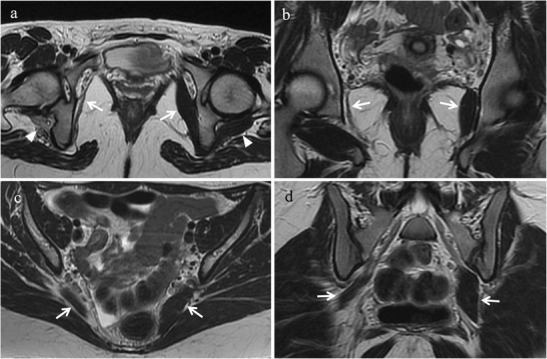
Indirect MR findings of sciatic nerve endometriosis. The same patient as in Fig. 23. (a) Axial and (b) coronal T2-weighted images show atrophy of right obturator internus (white arrows) and gemellus superior muscle (white arrowhead in a) compared with the contralateral ones (white arrows and white arrowhead in a). (c) Axial and (d) coronal T2-weighted images display atrophy of right piriformis muscle compared with the contralateral one (white arrows)
